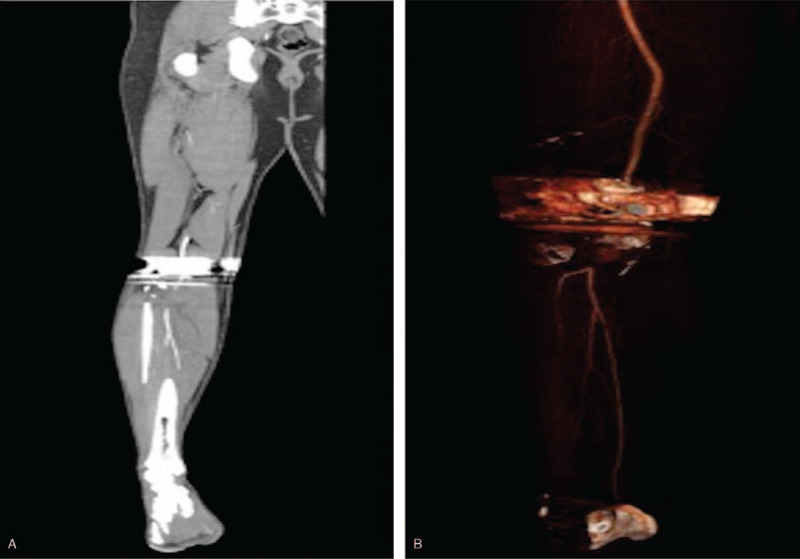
4A-B: Postoperative CT-A demonstrating a thrombus in the distal popliteal artery, inferior to the implant.
